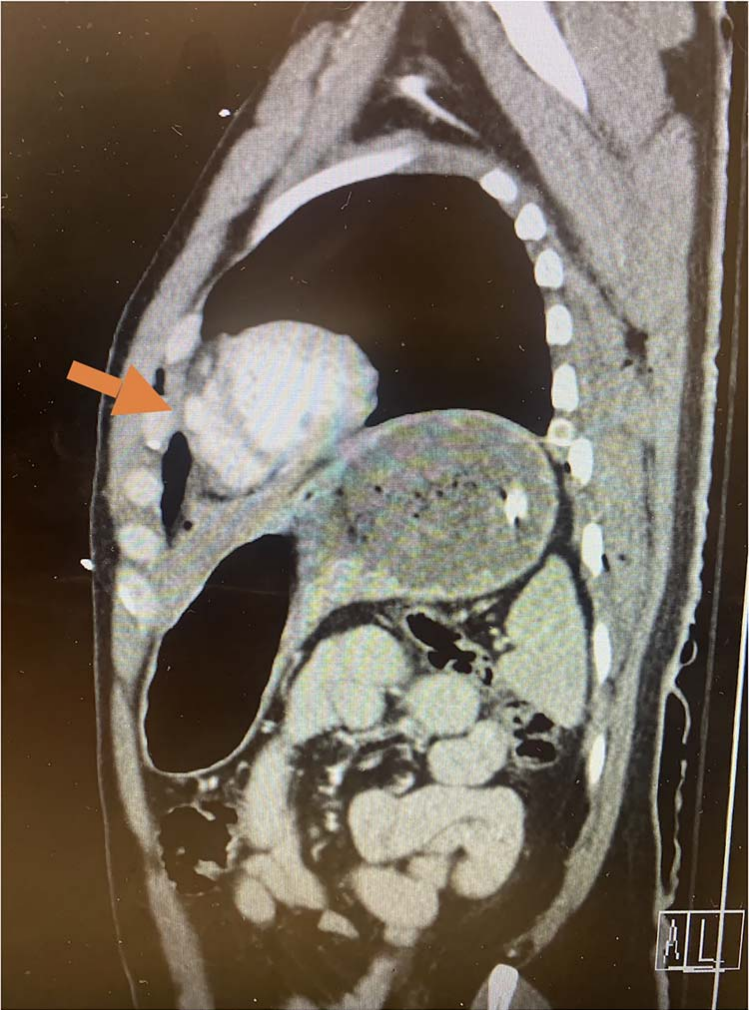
Sagittal CT imaging demonstrating pseudoaneurysm of right ventricular free wall rupture.
